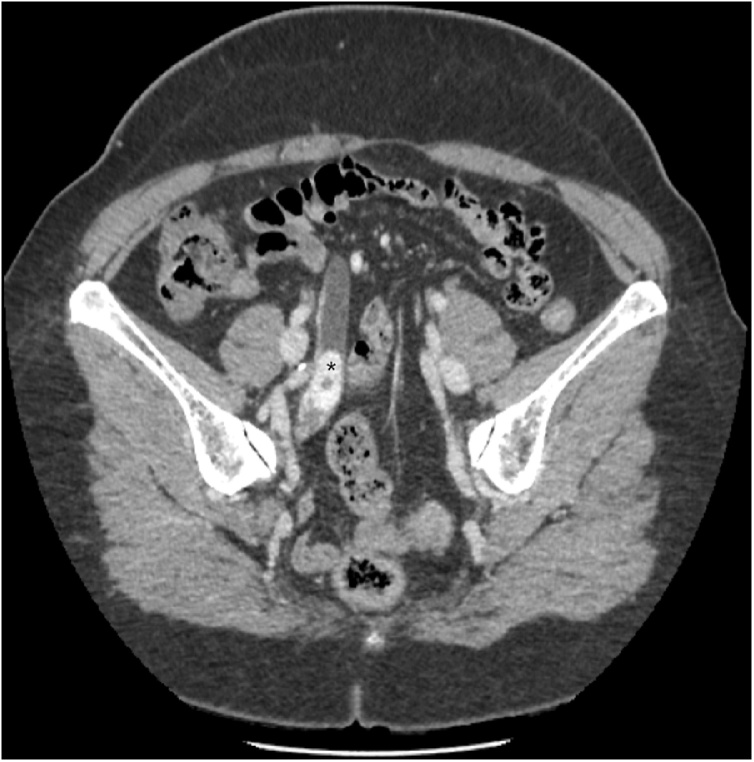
Oblique reconstruction late portal phase CT scan showing the tumor (asterisk) totally filling the right ureter.
